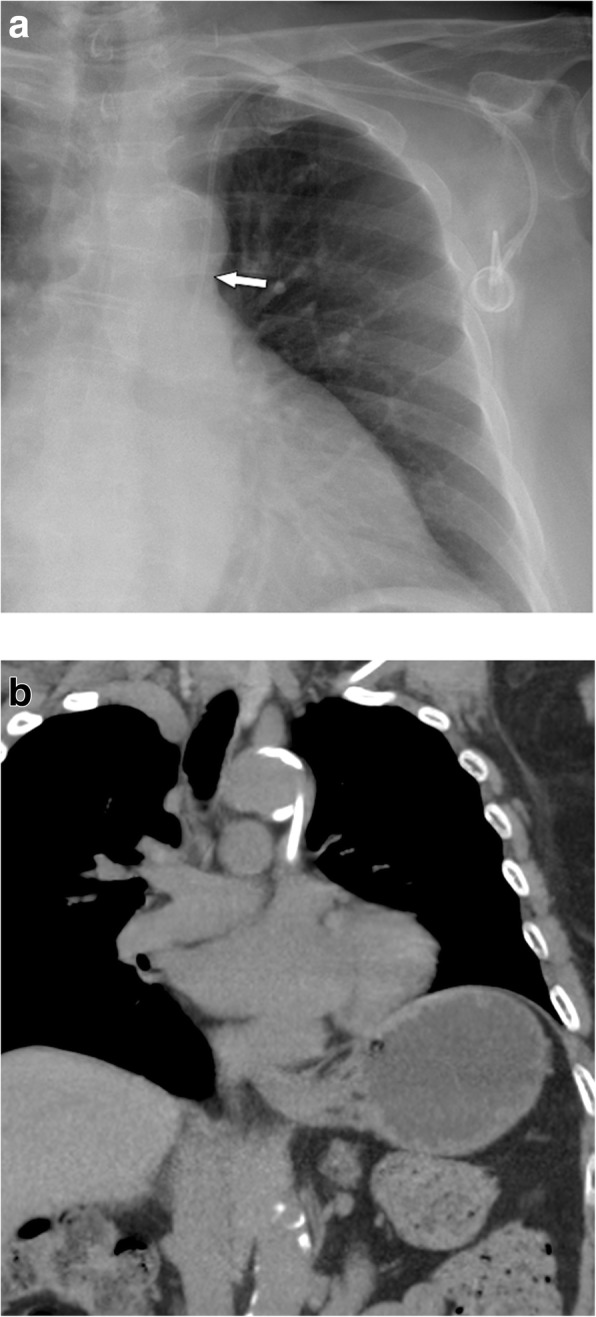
a Malpositioning in a persistent left superior vena cava: on a chest film, this could be confused with arterial malpositioning in the aortic arch via the left subclavian artery. b Unenhanced CT shows the tip of the catheter in the SVC, which drains into the right atrium
